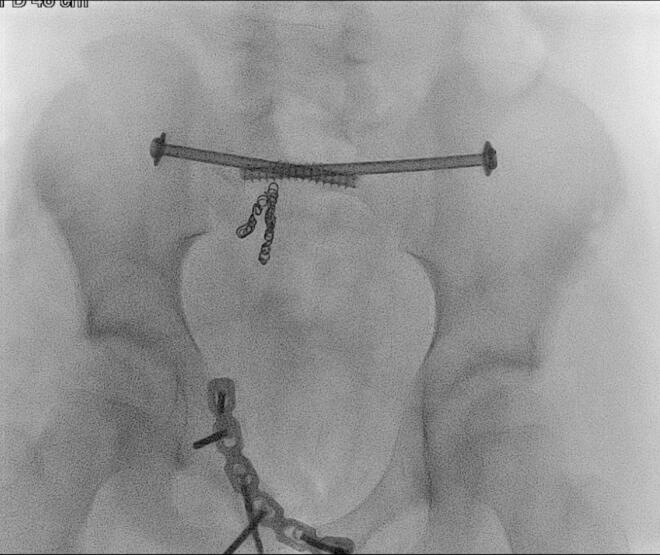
Per-operative inlet Pelvic radiograph during Open reduction and internal fixation.
